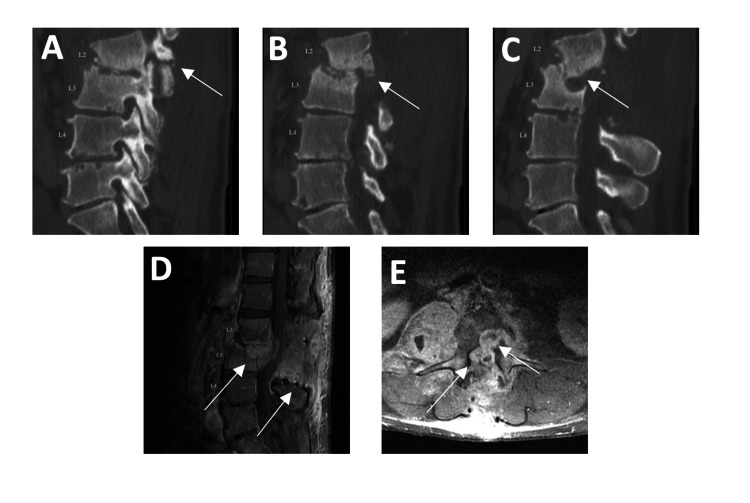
CT (A, B, and C) and MRI (D and E) of the lumbar spine taken during second rehospitalization. Significantly worsening kyphotic deformity with worsening L3 body destruction and now with retrolisthesis of L2 on L3 appreciated on both CT (A, B, and C) and MRI (D and E). MRI additionally demonstrates the extension of the abscess from the vertebral body to the canal resulting in moderate spinal canal stenosis and severe bilateral neural foraminal stenosis (D and E).
